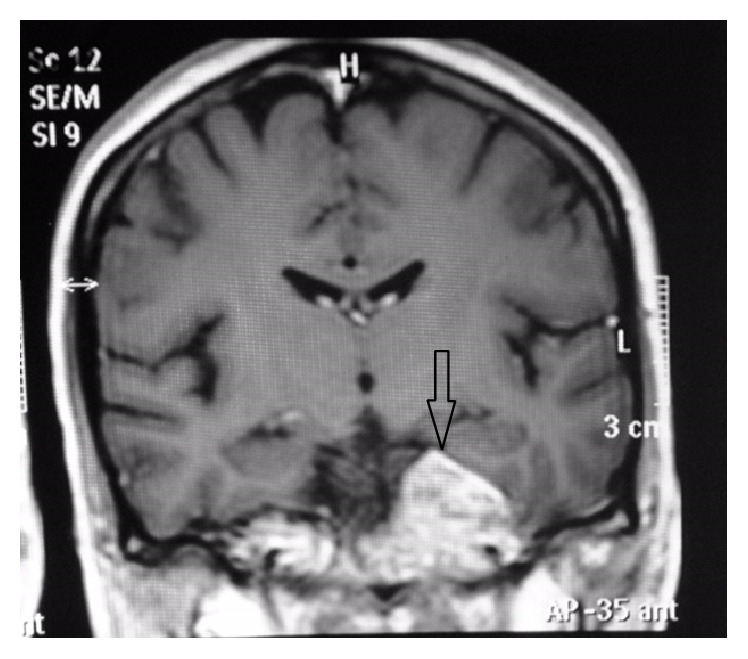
MRI precontrast showing altered signal intensity of the clivus, coronal section that appears hyperintense.
